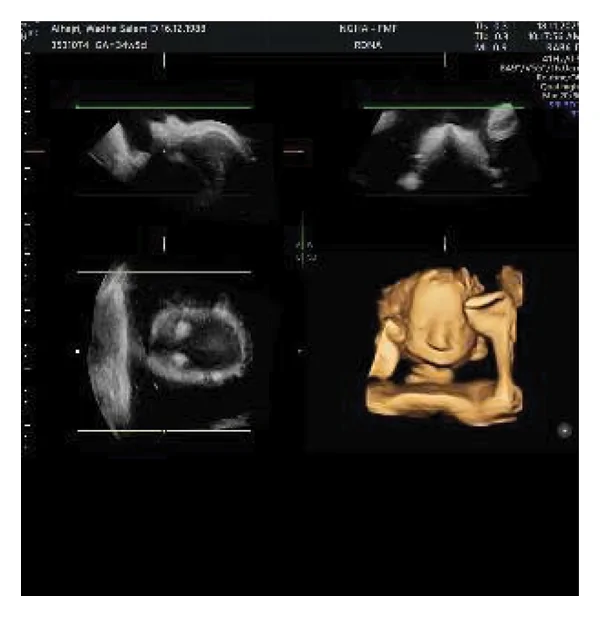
Prenatal three‐dimensional ultrasound imaging of severe micrognathia/agnathia.
